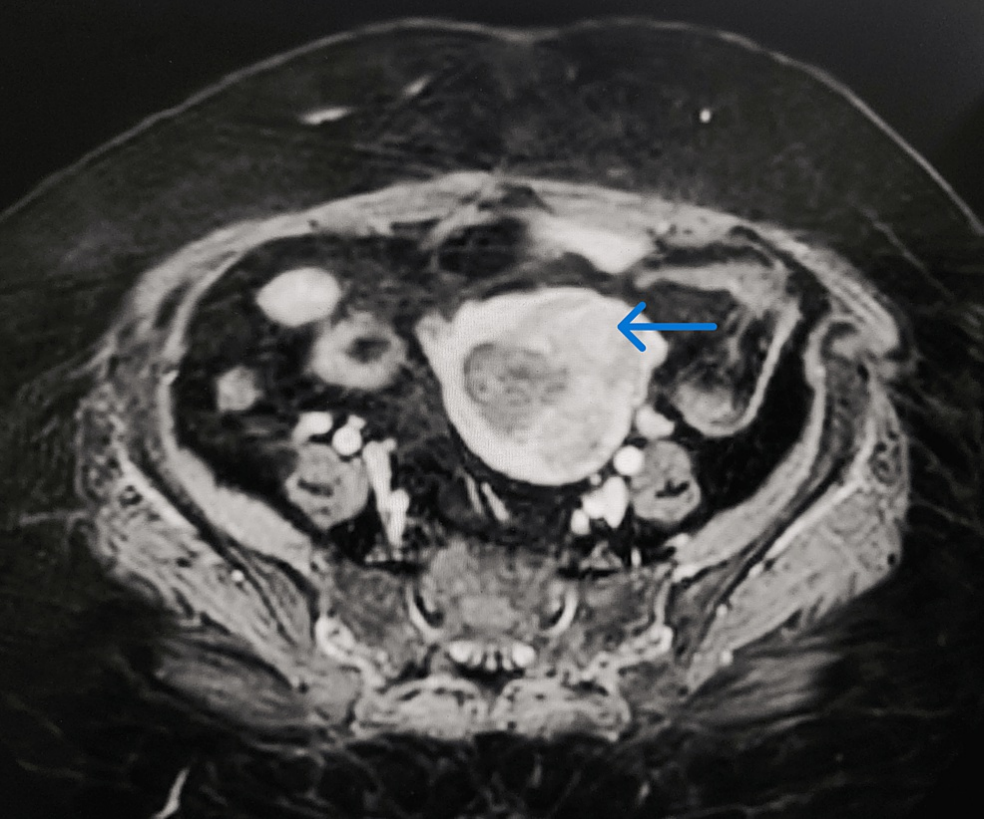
MRI-angiography confirmed the presence of a bulky uterus with heterogeneously enhancing lesion in the endometrial cavity with loss of endometrial-myometrial junction at the fundus and upper cornu on the left side. The lesion measured approximately 5.4 × 4.2 × 5.3 cm in side with a thickened endometrium of approximately 3 cm. MRI-angiography: magnetic resonance imaging with angiography
